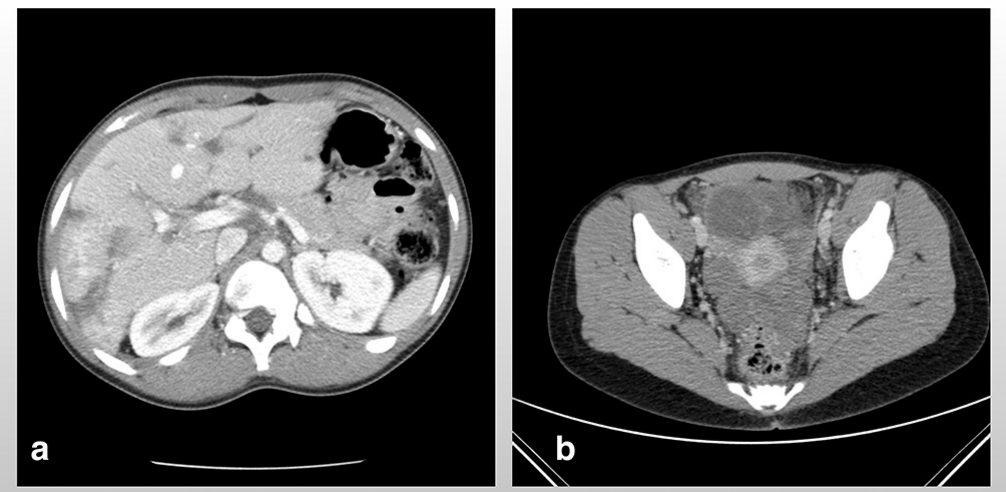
Axial CT scan of the abdomen and pelvis done approximately 1 year after 10 cycles of chemotherapy demonstrated a significant resolution of the hepatic lesions with residual low attenuation linear foci consistent with scarring and fibrosis (a). The pelvic mass showed a further significant reduction in size along with extensive low attenuation changes in keeping with necrosis. The uterus is now clearly visible surrounded by this pelvic mass (b).
